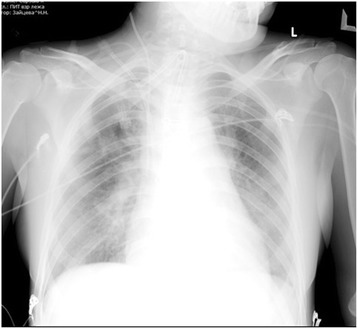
Chest X-ray: reperfusion pulmonary oedema after pulmonary thromboendarterectomy; Figure demonstrates the development of reperfusion pulmonary injury immediately after pulmonary thromboendarterectomy procedure
